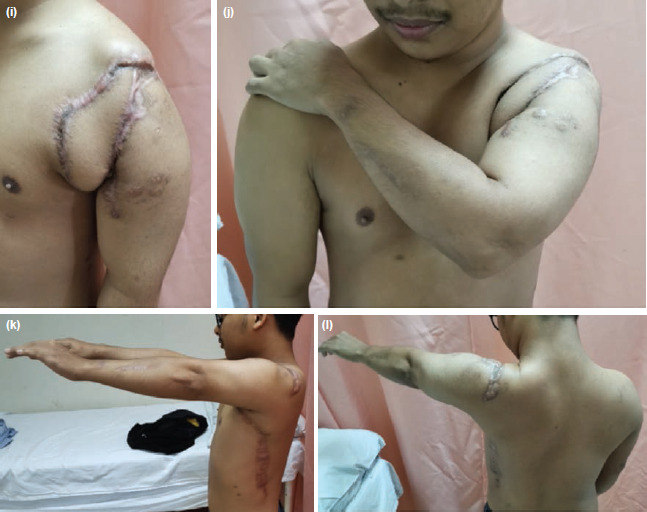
(a) Pre-operative planning of pedicled musculocutaneous latissimus dorsi flap. (b) The pre-planned pedicled musculocutaneous latissimus dorsi flap was raised. (c and d) Plain radiographs (AP and lateral view) showed there was no residual sequestrum after sequestrectomy. (e and f) Final plain radiographs in AP and lateral view. After completion of antibiotics and reducing septic parameters, a second-stage procedure was carried out, involving acute shortening, Proximal Humerus Internal Locking System (PHILOS) insertion, iliac bone grafting and pedicled musculocutaneous latissimus dorsi flap. (g) The pedicled musculocutaneous latissimus dorsi flap was used to cover the soft tissue defect of the shoulder. (h) Post-operatively, the flap appeared to be healthy. (i) Two years after the second stage surgery, the patient achieved both bony union and soft tissue recovery. (j) The shoulder adduction was good. (k) The patient was able to achieve forward flexion up to 90°. (l) The patient was able to achieve abduction up to 100°.
